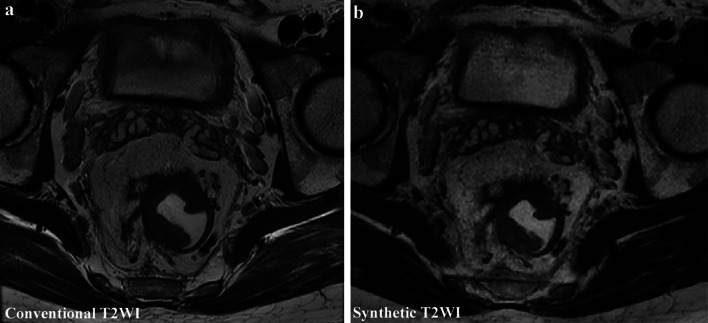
Conventional and synthetic T2-weighted imaging (T2WI) from a 46-year-old male patient with rectal cancer. Synthetic T2WI provided similar image quality with the conventional T2WI. The patient was evaluated as T3 stage with extramural venous invasion using both conventional T2WI and synthetic T2WI
